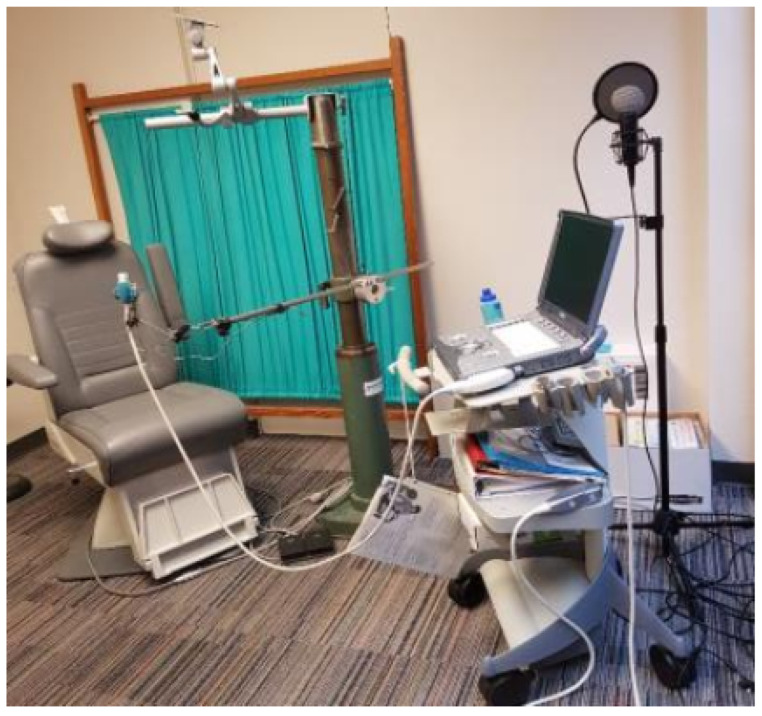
Ultrasound image acquisition system used in speech analysis. The system is also configured with a microphone and head-transducer stability system. The copyright for the ultrasound and head-transducer support system picture belongs to the author of this article, Khalid Al-hammuri [5].
